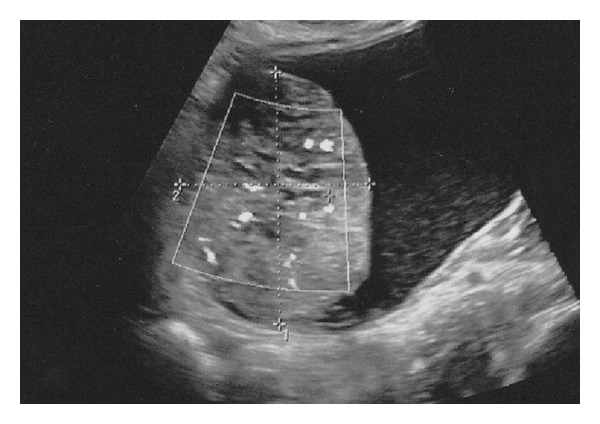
Ultrasound showing a complex mass, with a solid component.
